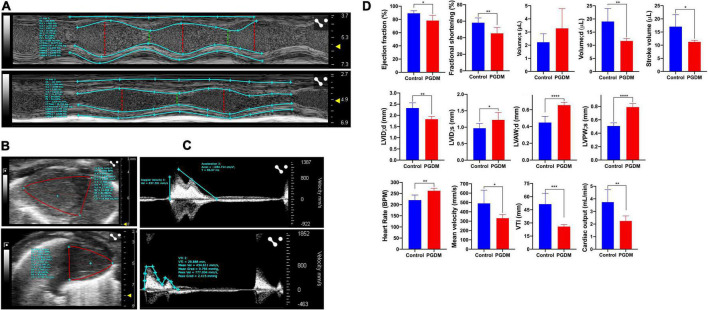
Echocardiography of the two-day neonatal hearts. Transthoracic echocardiographic analyses were performed using the Vevo LAZR-X ultrasound system: Representative images of M-mode (A), B-mode (B) and pulsed-wave doppler (C) traces of the left ventricles are shown, and different functional hemodynamic parameters were evaluated (D). Data are shown as Mean ± SD (*n* = 6), p* < 0.05, *p* < 0.01, p* < 0.001, *p* < 0.0001.
